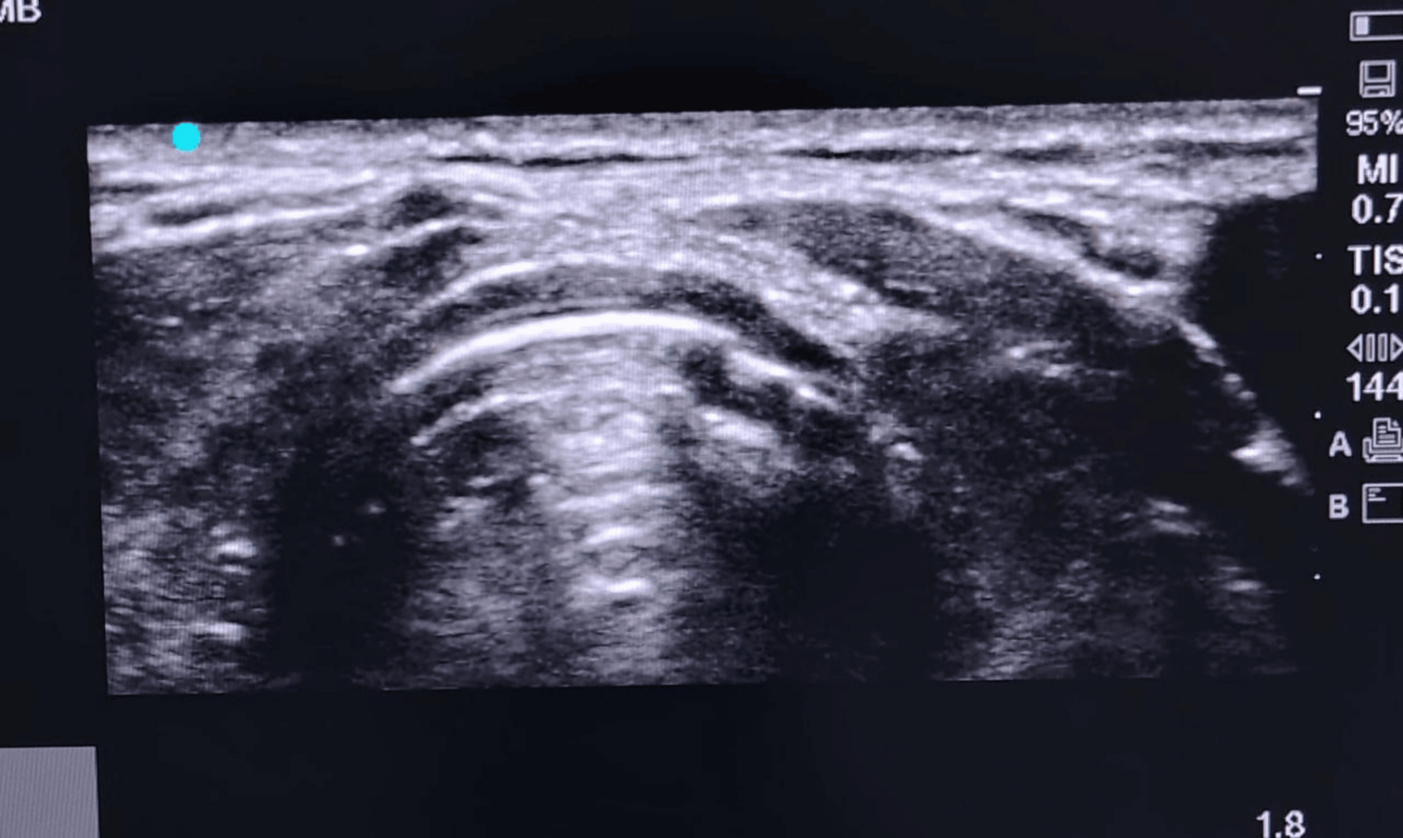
Suprasternal view The image demonstrates the trachea centrally, appearing as a hypoechoic rounded structure with posterior reverberation artifacts (comet tail), characteristic of air. The right and left thyroid lobes are seen laterally, appearing as homogeneous, moderately echogenic structures. The strap muscles are visualized anterior to the thyroid lobes as hypoechoic bands. This view is commonly used in thyroid and neck sonography for structural assessment. This original image was acquired directly from patients who participated in the study.
